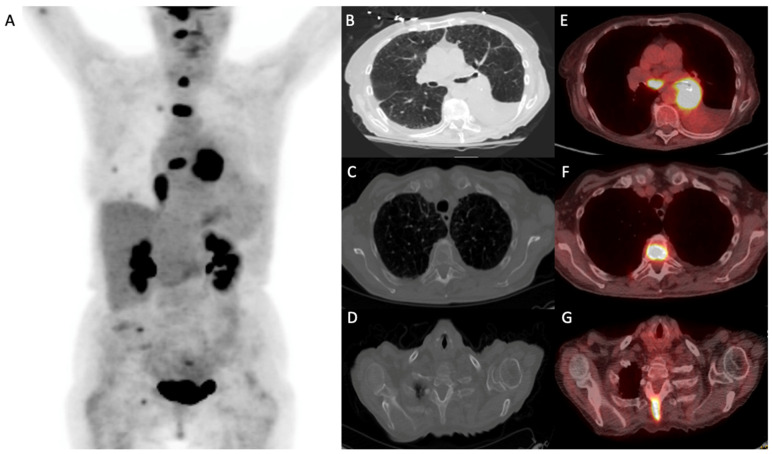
Demonstrates a 68-year-old man with biopsy-proven left lung squamous cell carcinoma (Stage: T4N2M1c). (A) ^18^F-FDG-PET/CT maximum intensity projection showing left lower lobe perihilar neoplasm invading the mediastinum, narrowing the left main bronchus with post-obstructive atelectasis; a biopsy demonstrated squamous cell carcinoma. (B–D) CT images and (E–G) fused images demonstrate enlarged FDG-avid subcarinal node suspicious for metastasis and multiple FDG-avid osseous lesions, with some lytic, and others CT-occult, consistent with metastases.
